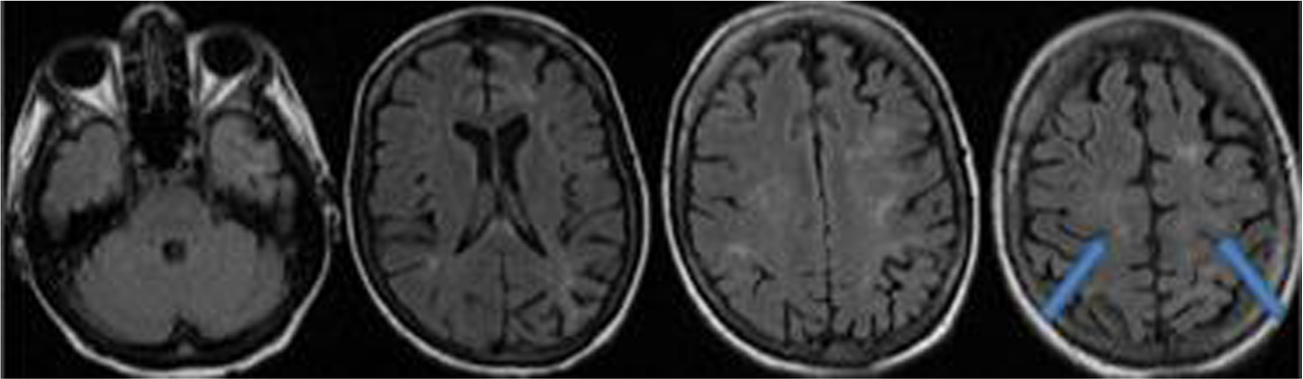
Case two, 2006: FLAIR MRI sequences demonstrating multiple poorly defined bright foci in the central and periventricular white matter, with subcortical U-fibre involvement (see *arrows*) in the frontoparietal region
